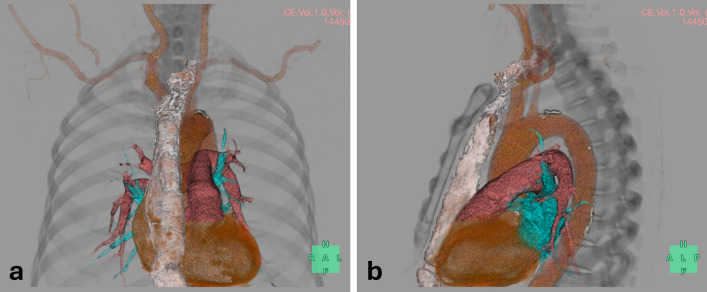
Three-dimensional reconstruction image from preoperative enhanced computed tomography. The spatial relationship between the retrosternal gastric tube (light pink) and heart is demonstrated. a Anterior view, b Left lateral view
